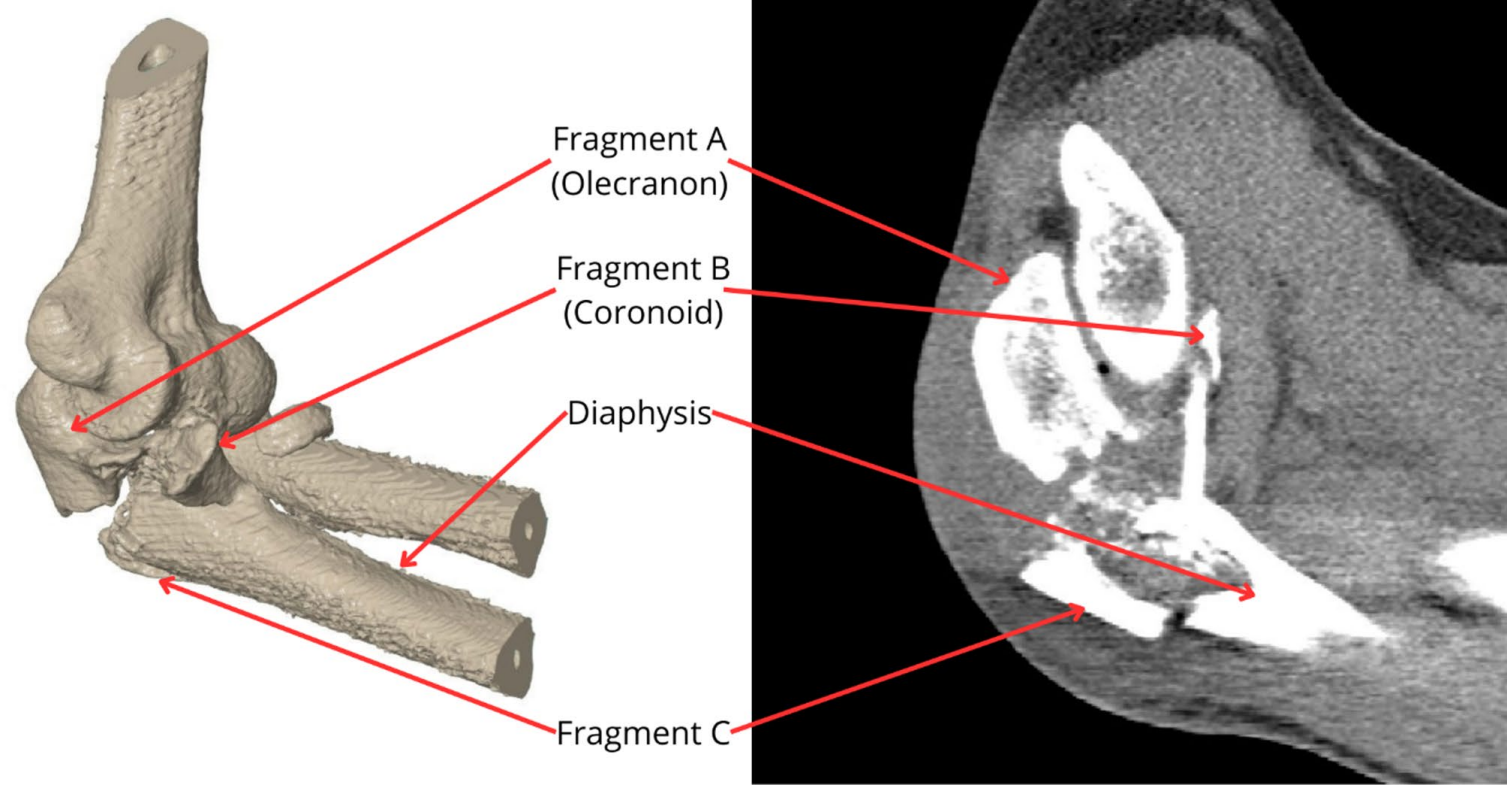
Analysing fracture of the ulna (left side– Model of the elbow joint with comminuted fracture; right side– CT image of the comminuted ulna)
